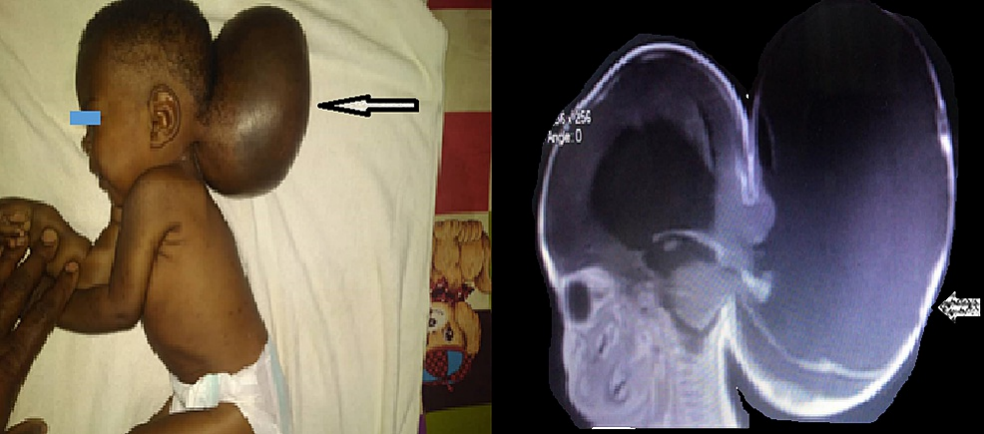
Pre-op evaluation of suboccipital cystic, transilluminating swelling with MRI; herniation of occipital and cerebellar lobes into a sub-occipital encephalocele with associated hydrocephalus.
